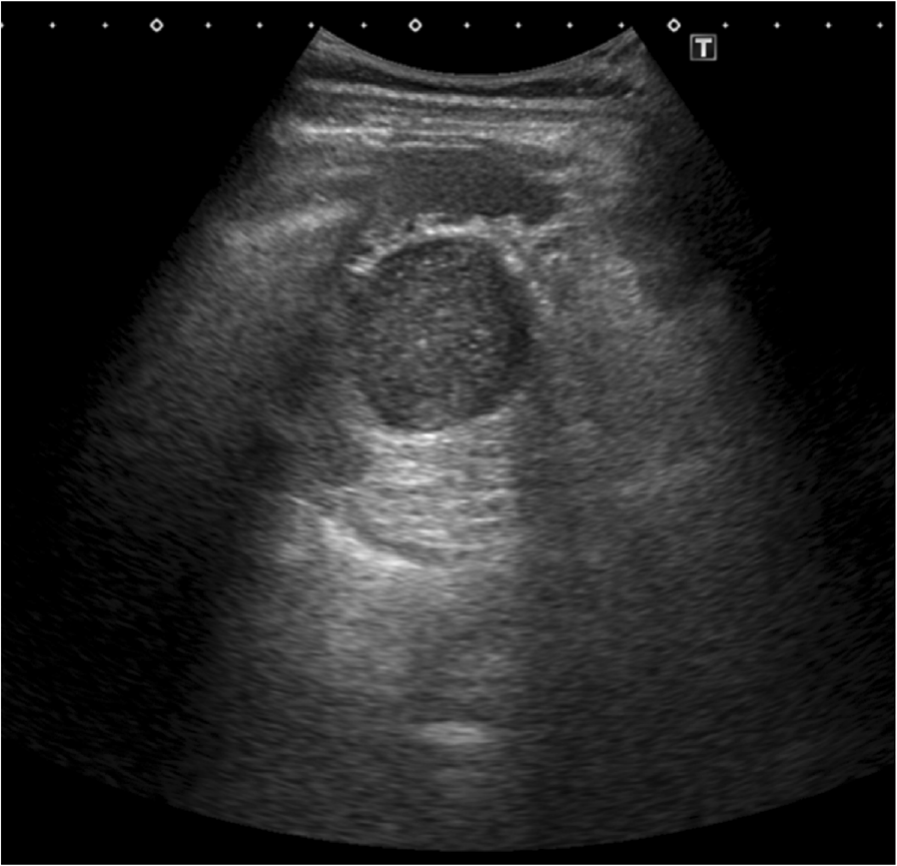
Abdominal ultrasound revealing a cystic lesion with a clearly defined boundary of approximately 40 mm in the pancreatic tail
